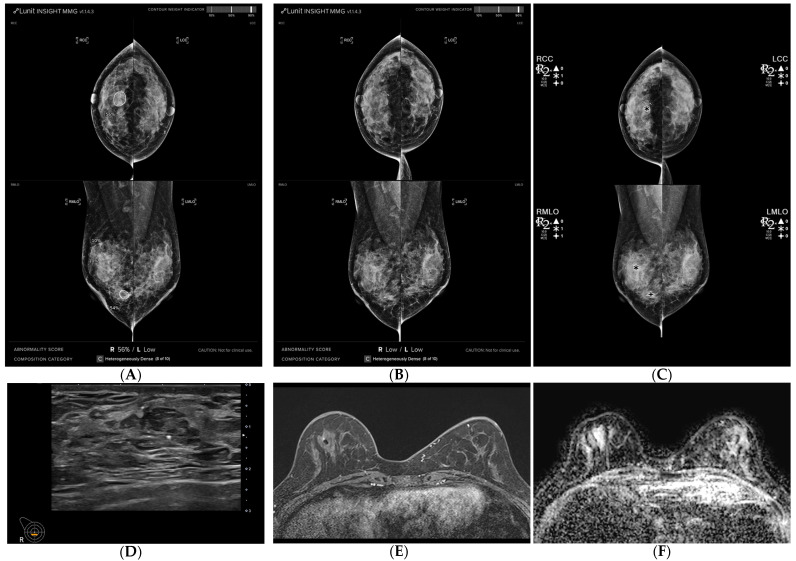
A 54-year-old woman diagnosed with breast cancer presenting as a mass with calcifications, exhibiting a surgically confirmed pathologic complete response (pCR) following neoadjuvant chemotherapy (NAC). (A,B) Mammography was analyzed using AI-CAD, identifying an area in the left central breast of a mass with calcifications in pre-NAC images (AI-CAD score 98% on the MLO view), and no mark was detected in post-NAC images. (C) Utilizing conventional CAD, identifying an area in the left central breast corresponding to the calcifications in post-NAC images. (D) Post-NAC ultrasound recorded a reduction in mass size from 26 mm. (E) Post-NAC maximal intensity projection (MIP) of contrast-enhanced MRI (CE-MRI) documented a mass reduction from 36 mm. (F) Post-NAC ADC map of DWI displayed regression of the mass.
